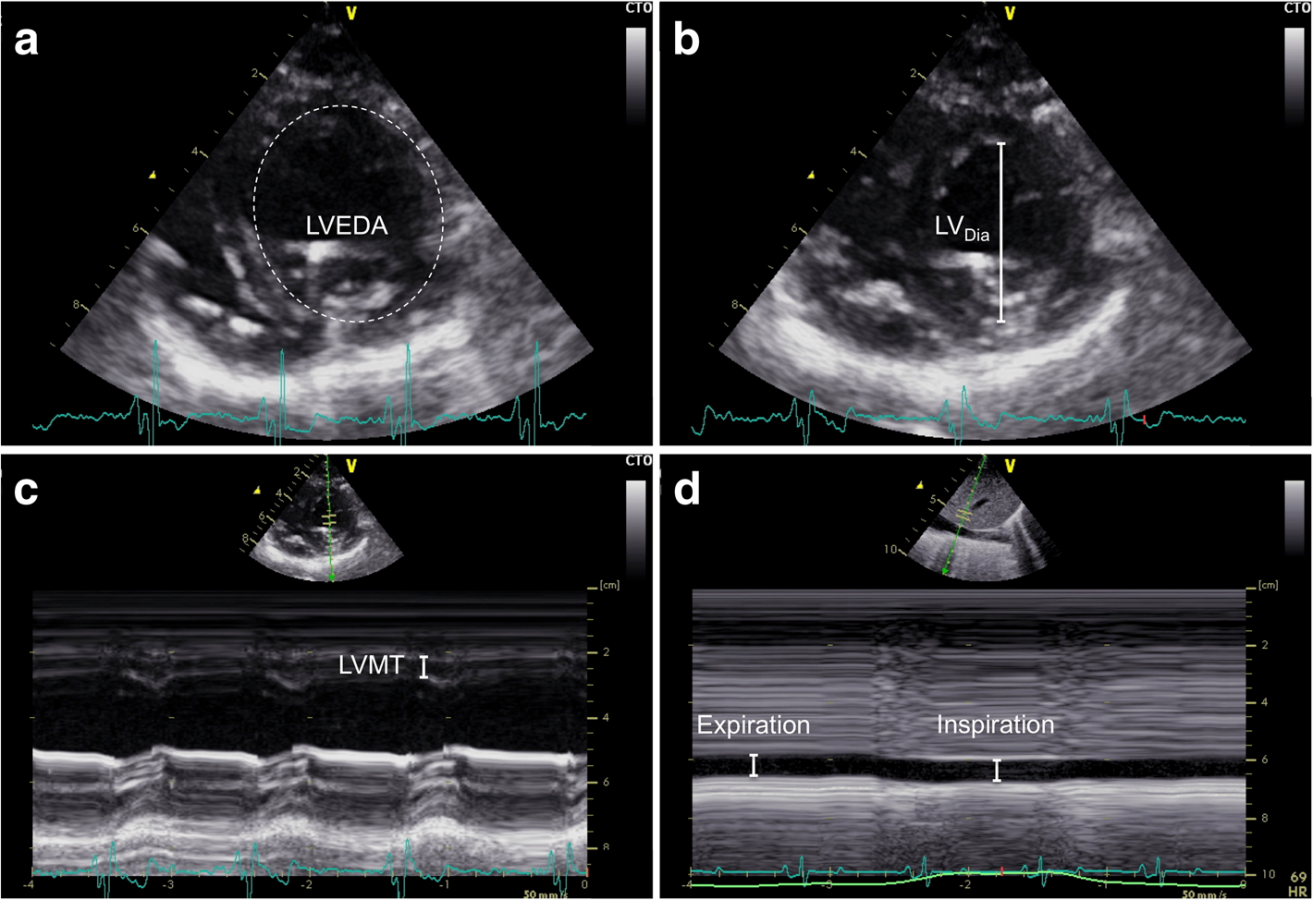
Ultrasonographic data acquisition. Ultrasonographic images of the left ventricle obtained from a parasternal short-axis view. Endocardial tracing allowed for measurement of left ventricular end-diastolic area (LVEDA) (a), left ventricular systolic diameter (LV_Dia_) (b) and left ventricular myocardial thickness (LVMT) (c) to enable afterload calculation. Inferior vena cava diameter was measured with anatomical M-mode at expiration and inspiration (d) guided by a respiratory tracing curve (green)
